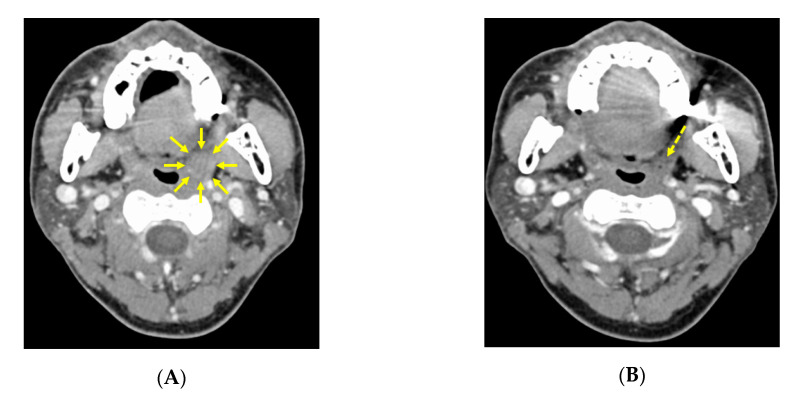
CT imaging findings of the primary tumor before and after neoadjuvant chemotherapy. (A) Pre-chemotherapy CT scan showing the left oropharyngeal lateral wall with the primary tumor is larger than the right side. The tumor is about 15 × 15 mm (arrows). (B) CT scan after the second neoadjuvant chemotherapy course showing a markedly smaller remnant left tonsil mass.
